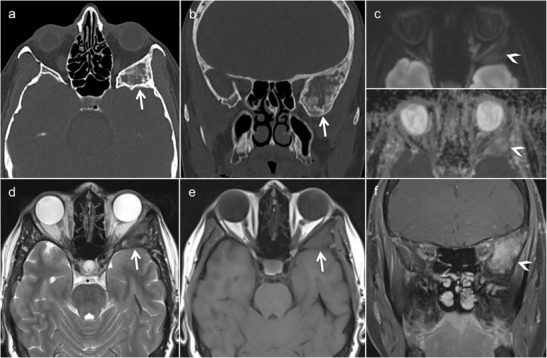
Magnetic resonance imaging (MRI) findings of FD involving the greater wing of sphenoid bone in a patient with left orbital pain. Axial (a) and coronal (b) CT images (bone window) show an enlarged greater wing of the left sphenoid bone (*arrows*) with medullary expansion and intact cortical outline. Ground-glass opacities within the affected bone. No bone destruction. c Diffusion-weighted imaging ((DWI) reveals intermediate signal on the b = 1000 image (*arrowhead*, *upper image part*) and on the ADC map (*arrowhead*, *lower image part*, apparent diffusion coefficient [ADC] = 1.4 × 10^−3^ mm^2^/s). d Heterogeneous low signal on T2 without intracranial extension. e Homogeneous low T1 signal (*arrow*). f Heterogeneous non-specific enhancement on the gadolinium-enhanced coronal fat-saturated T1 (*arrowhead*)
